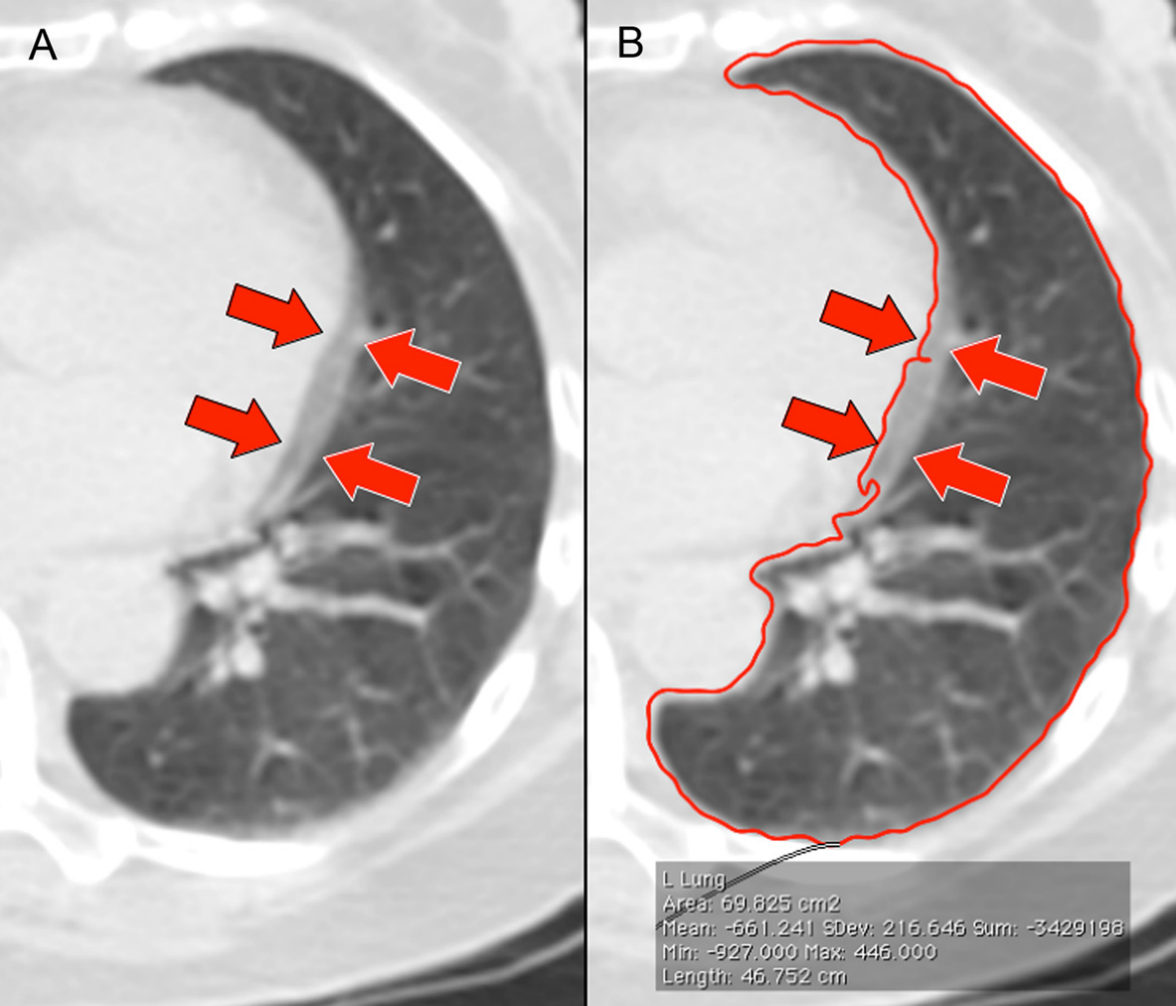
Cardiac motion artefacts on SPECT/CT. Cardiac motion artefacts were frequently observed at the left mediastinal border (arrows) (A), often leading to auto-segmentation errors (red contour line) (B) requiring manual correction.
